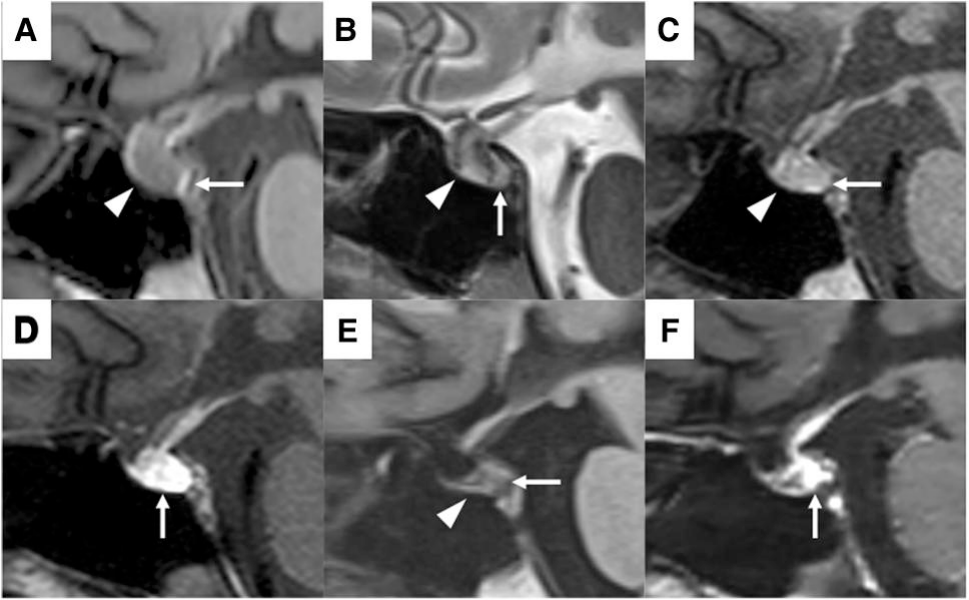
Sagittal MR image of the pituitary. A, T1WI on day 6. The anterior lobe is swollen with a diffuse low signal (arrowhead), and a normal high signal of the posterior lobe is clearly demonstrated (arrow). B, T2WI on day 6. The anterior lobe is demonstrated as an inhomogeneous high signal (arrowhead). The posterior lobe is also demonstrated as a high signal (arrow). C, T1WI on day 12. The swelling of the anterior lobe is improved (arrowhead), and the high signal of the posterior lobe is absent (arrow). D, Contrast enhancement on day 12. The posterior part of the anterior lobe and the whole posterior lobe are enhanced (arrow). E, T1WI on day 45. The anterior lobe is atrophic (arrowhead), and the high signal of the posterior lobe remains absent (arrow). F, Contrast enhancement on day 45. The contrast enhancement in the posterior part of the pituitary lobe is shrunk (arrow).
